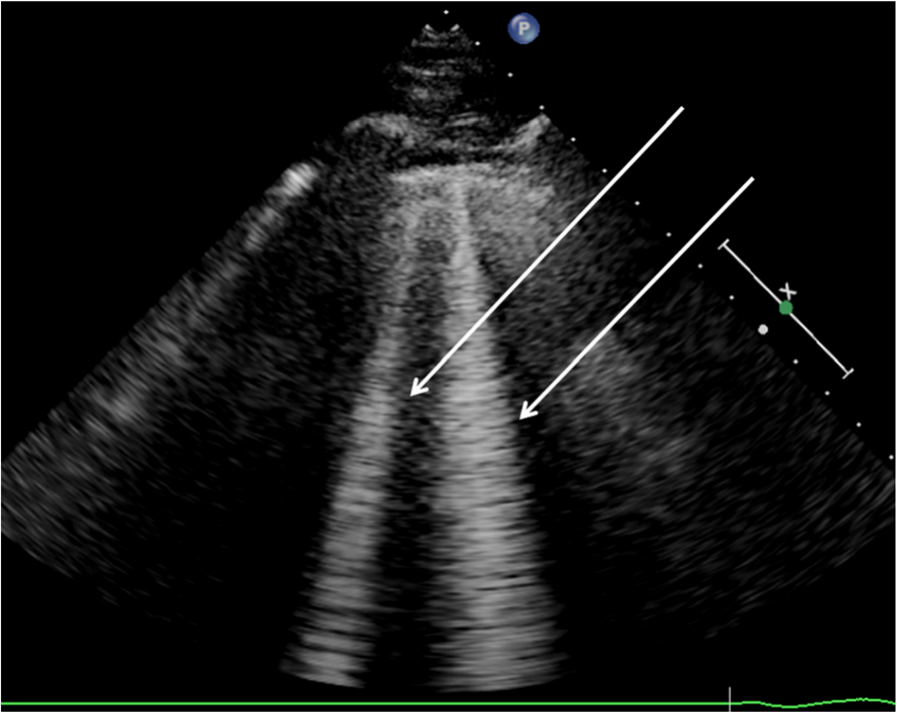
Two ultrasound lung comets (arrows) arising from the pleural line
